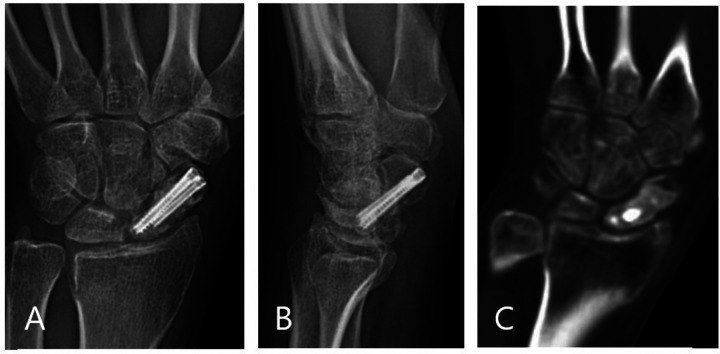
Anteroposterior (A) and lateral (B) x-ray and coronal CT scan (C) confirming the solid union of the fracture site.
